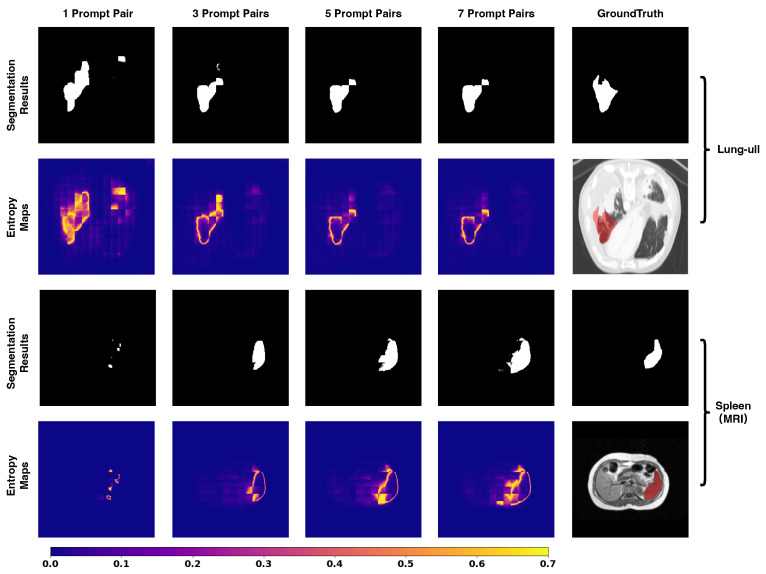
Visual results for lung lobe (CT) and spleen (MRI) segmentation. While the segmentation performance steadily improves with an increasing number of prompts, the prediction maps still exhibit high entropy values.
